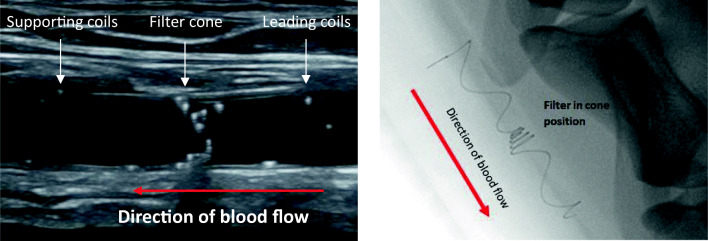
Ultrasound (left panel) and X-ray (right panel) images of implant
